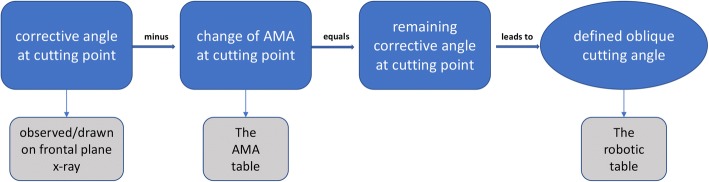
Calculation for clinical practice: Processing radiographically observed corrective angle and change of AMA to the remaining corrective angle, which leads to a defined oblique cutting angle
